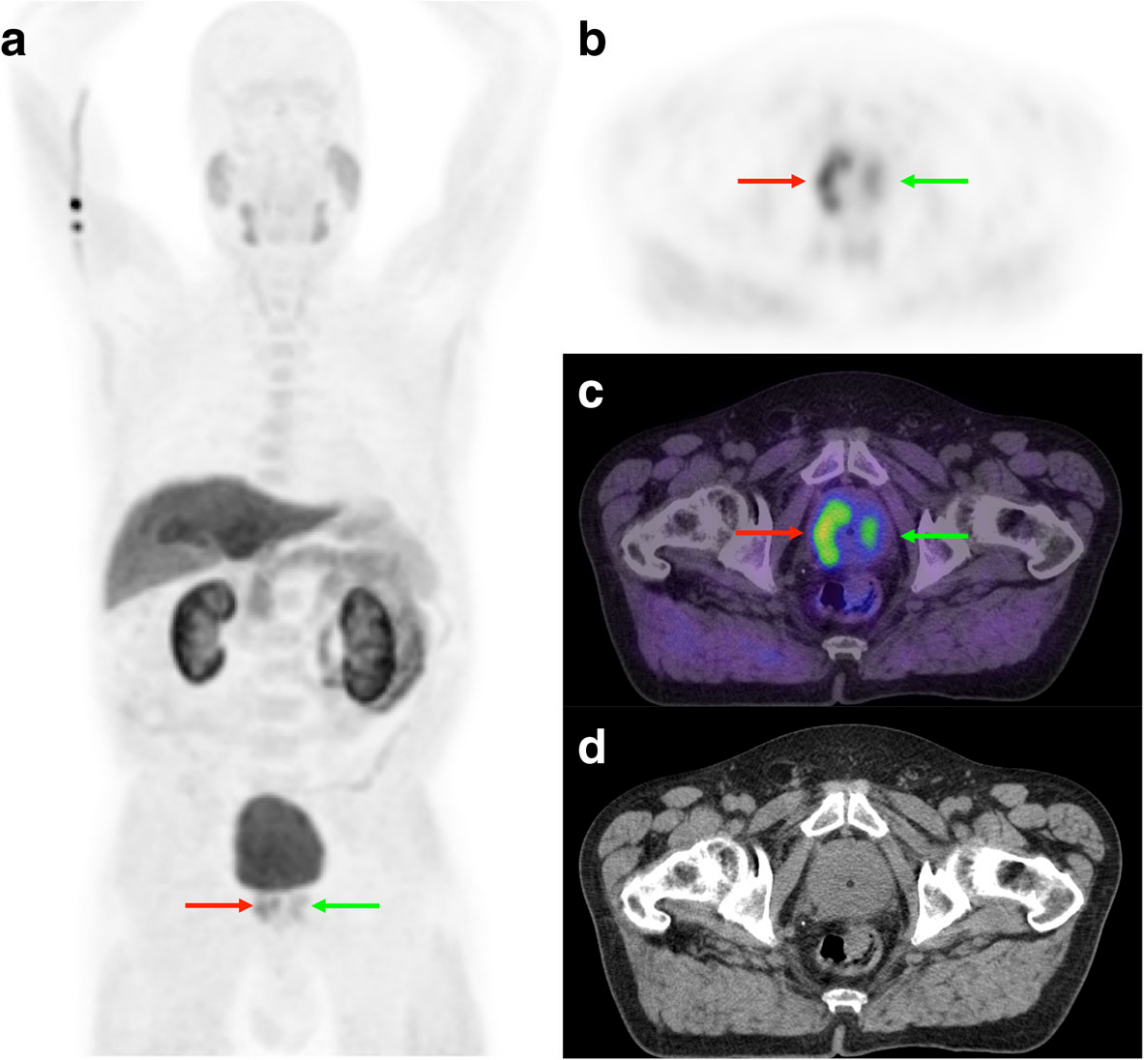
FCH-PET/CT for staging and radiotherapy planning in a 78 y.o. patient with biopsy-proven PCa. Biopsy Gleason sum was 7 and PSA was 13 mg/L. MIP (a) and transaxial PET (b), fusion (c) and CT (d) views showed a moderately FCH-avid, multi-focal dominant intra-prostatic lesion in the right prostatic lobe (red arrows), consistent with a primary PCa lesion and the biopsy findings. A second area of milder focal uptake was seen in the left prostatic lobe (green arrows) and raised some suspicion for a contralateral PCa lesion. However, both the initial biopsy and a repeat biopsy were negative for the presence of PCa in that area, and the patient received high dose-rate brachytherapy to the right dominant lesion only, in addition to external radiotherapy
